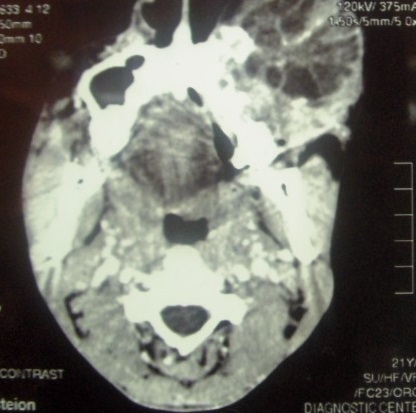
Axial section CT scan showed left maxillary expansion with mixed radiolucency
